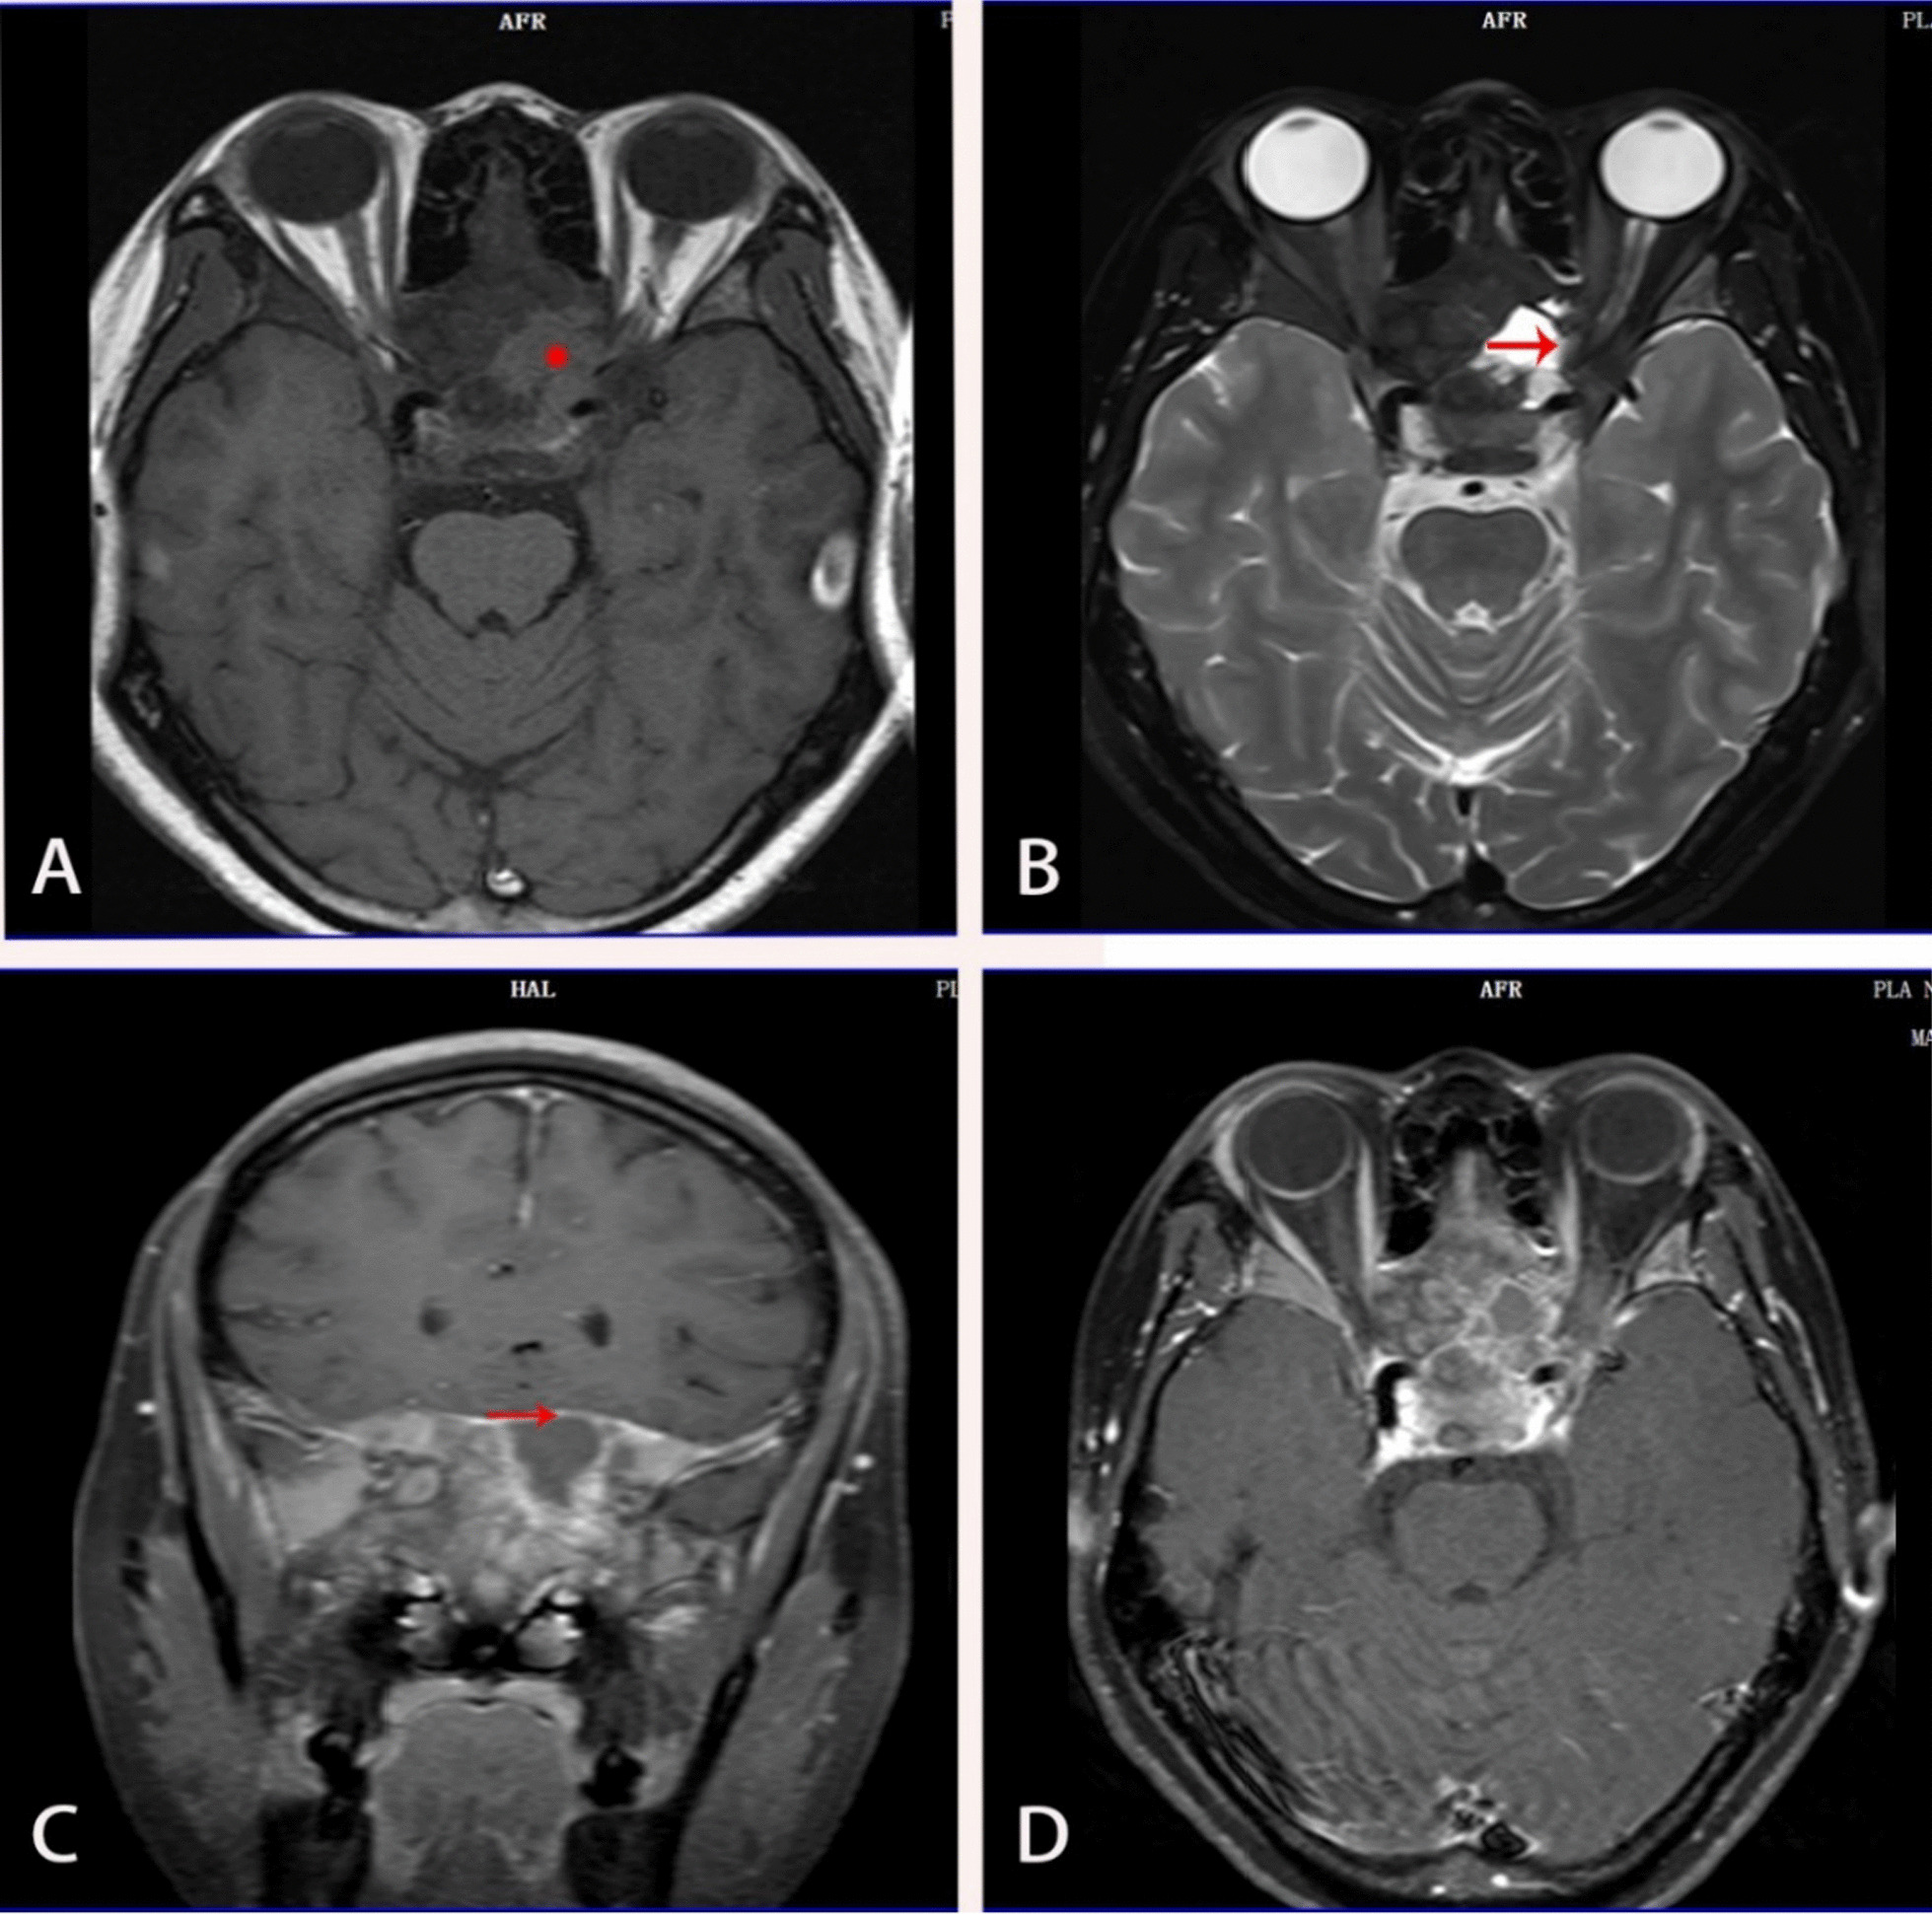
Orbital MRI before surgey. A Orbital MRI indicates cystic degeneration in patients with fibrous dysplasia of bone (marked by red stars). B The red arrow shows the optic nerve in the canal. C The red arrow shows the integrity of the dura mater and no collapse of the skull base. D Shows the overall situation in MRI
